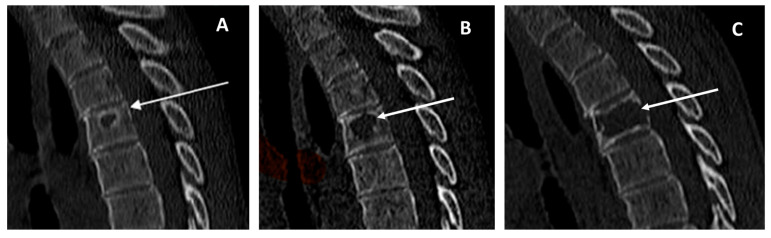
CT scans (sagittal view) in a female patient with a diagnosis of adamantinoma at the age of 26 years old. Chest CT-scan performed 8 months after diagnosis (panel (A)) revealed a geographic lytic lesion with sclerotic borders within the T4 vertebral body (arrow). The lesion was initially misdiagnosed due to the non-aggressive radiological appearance (Lodwick–Madewell grading 1A). The lytic lesion showed progressive dimensional increase over time in subsequent CT follow-ups (panels (B,C), arrows). A CT-guided biopsy revealed an adamantinoma metastasis.
